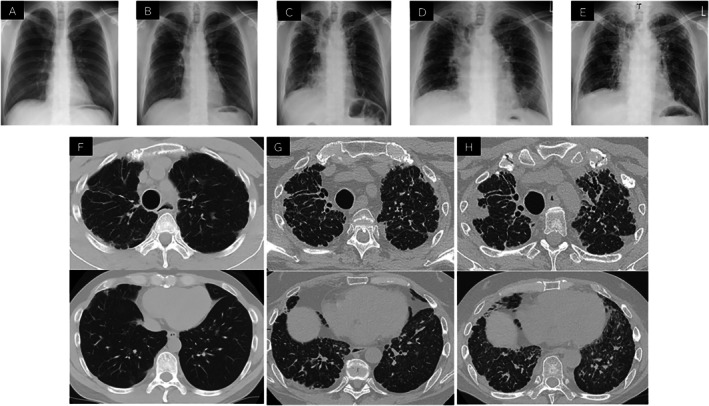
Time course of chest x‐ray and computed tomography findings. (A) No apparent abnormalities were observed on chest x‐ray at the time of PBSCT. (B) Three years after transplantation, chest x‐ray revealed mild bilateral upper lung field–predominant subpleural opacities. (C) Ten years after transplantation, at referral to our hospital, subpleural infiltrative opacities predominantly in the bilateral upper lung fields with marked volume loss and rightward tracheal shift were noted, along with reticular opacities and volume loss in the bilateral lower lung fields, predominantly on the right. (D) At 11years post‐transplantation and one year after referral to our hospital, further progression of rightward tracheal deviation was noted, along with newly developed infiltrative opacities in the left lower lung field. (E) At 11 years post‐transplantation and 2 years after referral to our hospital, chest x‐ray obtained during an acute exacerbation triggered by SARS‐CoV‐2 infection revealed newly developed bilateral ground‐glass opacities and pneumomediastinum. (F) Five years after transplantation, chest CT revealed linear lesions arising from the subpleural regions predominantly in the bilateral upper lobes, accompanied by atelectatic consolidation. (G) Ten years after transplantation, at the time of referral to our hospital, chest CT showed progression of linear lesions and atelectatic consolidation in the bilateral upper lobes, accompanied by traction bronchiectasis and marked volume loss. In addition, granular opacities and interlobular septal thickening were observed predominantly in the right lower lobe. (H) Twelve years after transplantation and 2 years after referral to our hospital, chest CT demonstrated further progression of traction bronchiectasis and atelectatic consolidation in the bilateral upper lobes, with marked volume loss. In addition, patchy ground‐glass opacities were newly observed in both lungs.
